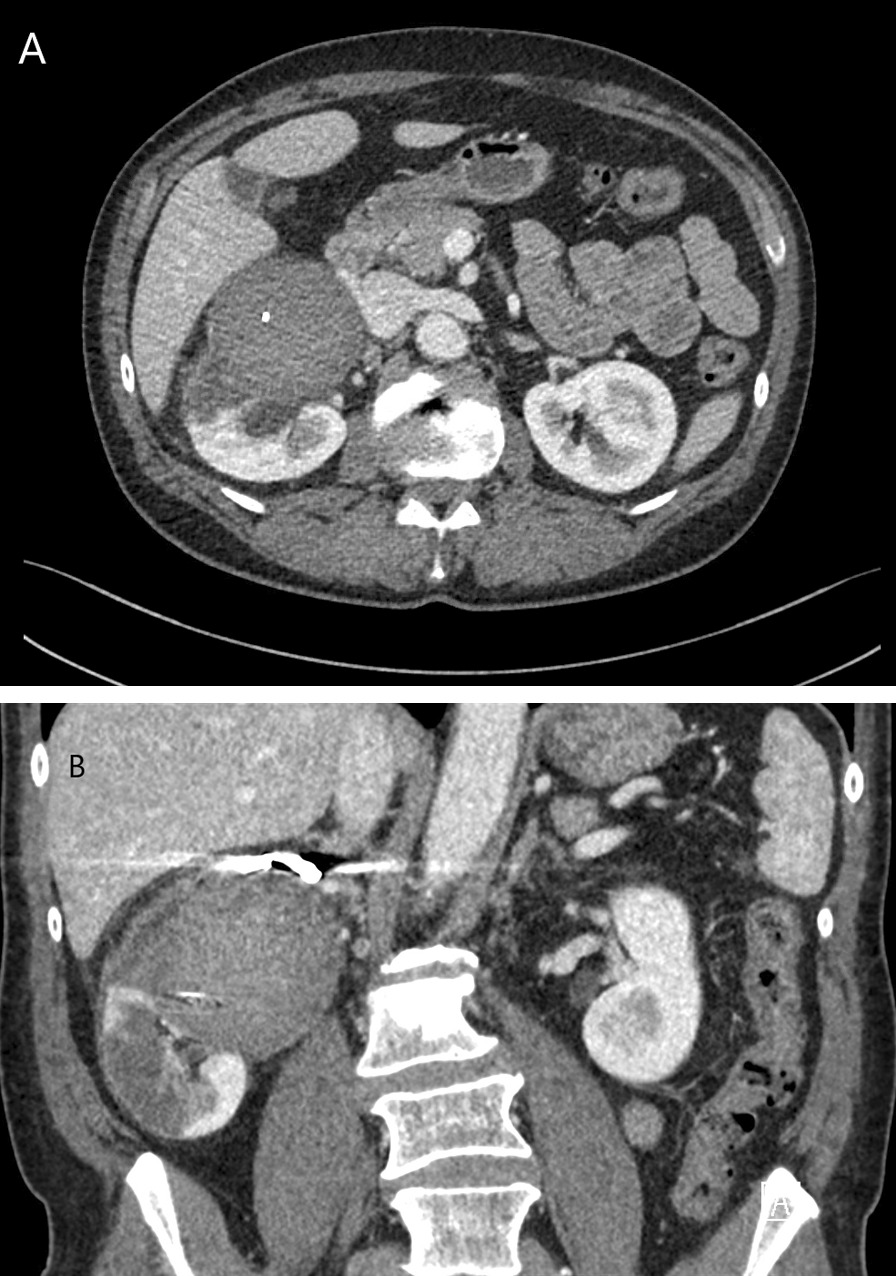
CTA (a, b) performed on postoperative day 14 demonstrated a 7.5 cm aneurysm with no contrast enhancement on arterial or delayed phases of imaging. There was hypoperfusion of the superior, anterior, and lateral portions of the right kidney indicating renal infarct
